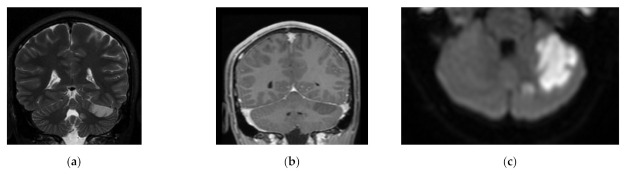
Focus of POCI (posterior circulation infarct) in the left SCA/AICA (superior cerebellar artery/anterior inferior cerebellar artery) territory in left cerebellar hemisphere in acute stage on the day of onset: (a) T2 MR; coronal plane; (b) T1 + CE coronal plane; (c) DWI MR—axial plane—restricted diffusion in the region.
